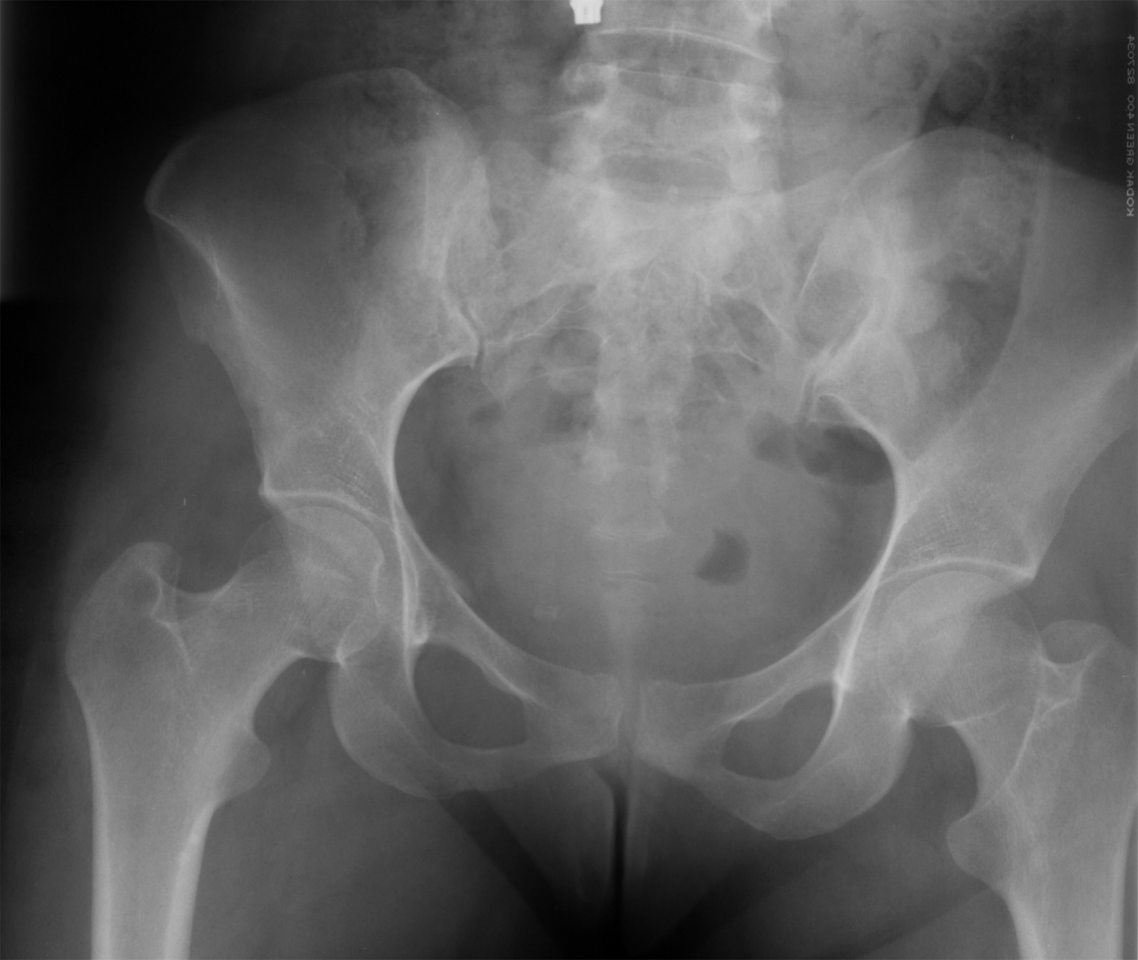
A vertical fracture line on the S1 vertebra can not be seen clearly on plain radiograph.
